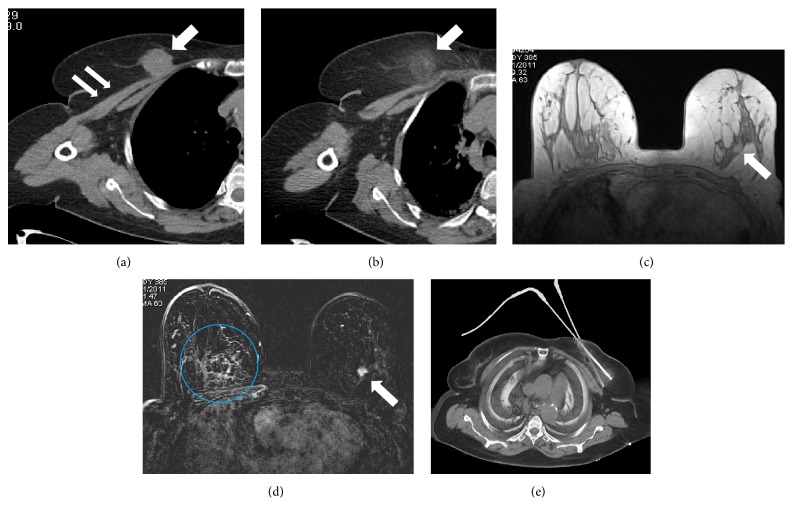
CT scan of an 81-year-old woman using the mediastinal window setting showed a primary breast cancer of the right breast (arrow) infiltrating the major pectoralis muscle (double arrow) (a). The patient received cryoablation of the primary tumor. CT scan obtained at the end of the cryoablation procedure showed the presence of a homogeneous area of low density because of the iceball (arrow), which encompassed the tumor (b). The same patient developed a contralateral breast cancer after 13 months. T2-weighted MR image showed a breast tumor in the left breast (arrow) (c). Contrast-enhanced T1-weighted fat suppression MR image showed the complete ablation of the cancer of the right breast and the contralateral tumor (circle and arrow, resp.) (d). CT-guided cryoablation of the left breast cancer using two cryoprobes (e).
